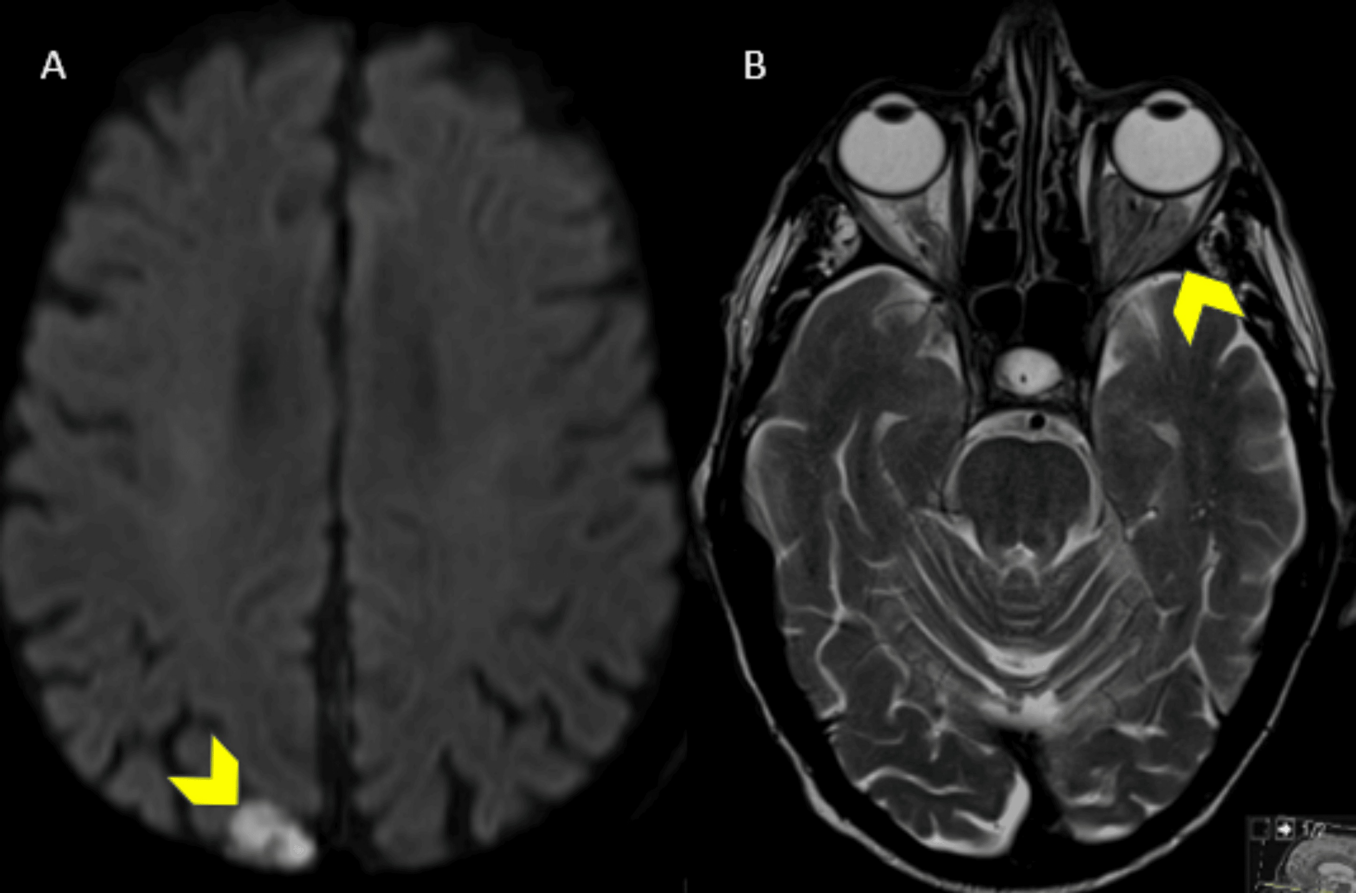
Axial diffusion-WI (A-DWI) and T2-WI MRI shows restriction of the lesions (arrowhead in A) and low T2 signal (arrowhead in B), which demonstrates their high cellularity MRI: magnetic resonance imaging
